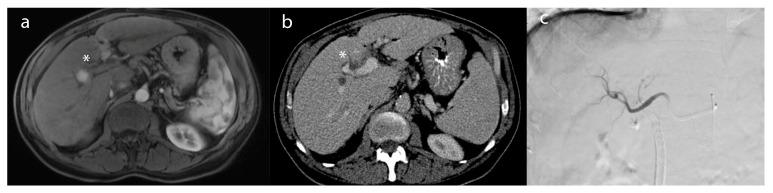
A 62-year-old female patient with (a) an arterially-enhancing lesion (*) in segment 8, compatible with hepatocellular carcinoma on post-contrast T1-weighted imaging. (b) After TACE, the lesion (*) demonstrated a lack of enhancement, compatible with a complete radiographic response on the 2 month follow-up CT. (c) Intraprocedural angiogram of TACE depicting the embolic distribution of the right lobar artery.
